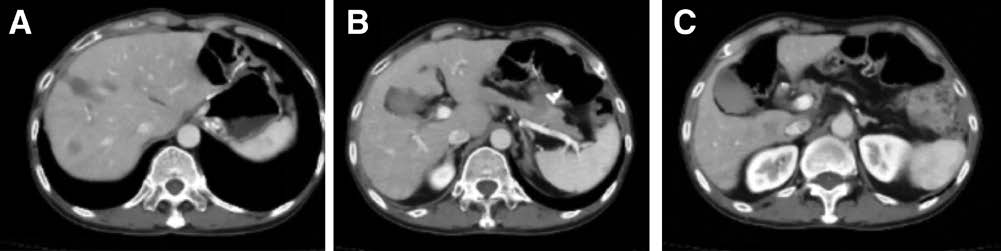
Subsequent CT examination (April 7, 2023) showed that compared to the old film, the nodules in the S7 and S6 segments of the liver were reduced; reduction of nodules at the junction of the pancreatic body and tail; there is a decrease in perihepatic fluid accumulation and a small amount of pelvic fluid accumulation, with no significant changes observed. CT = computer tomography.
